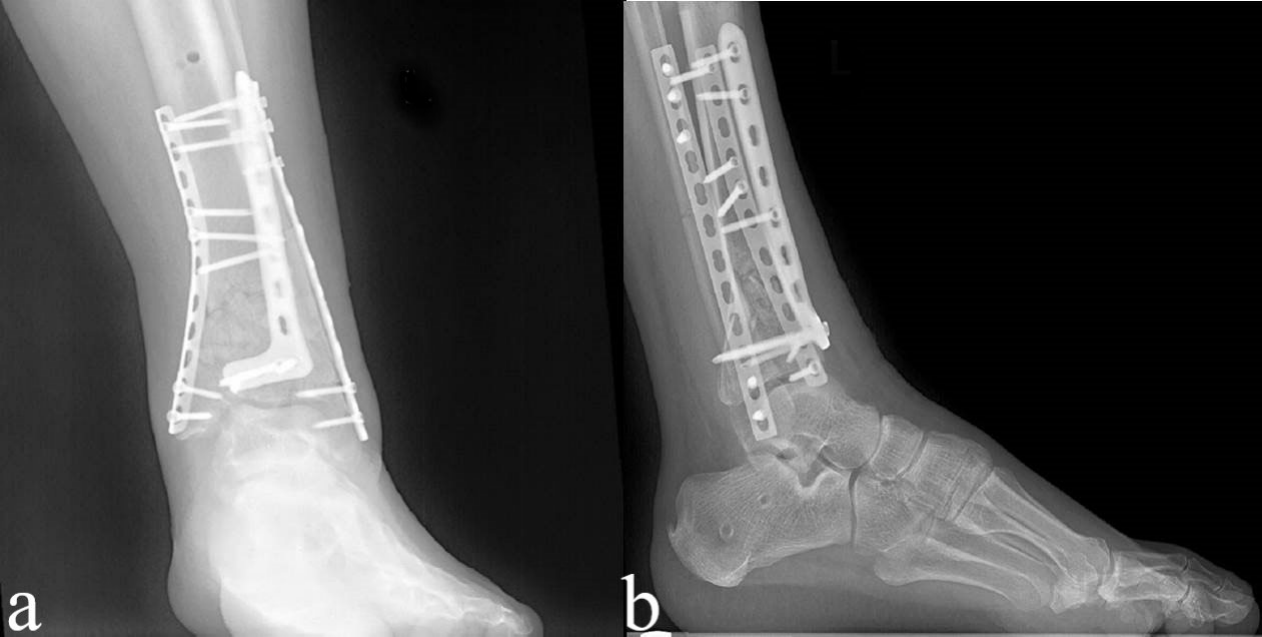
Anteroposterior (a) and lateral (b) x-ray images after ORIF (second step of two stage surgery).
